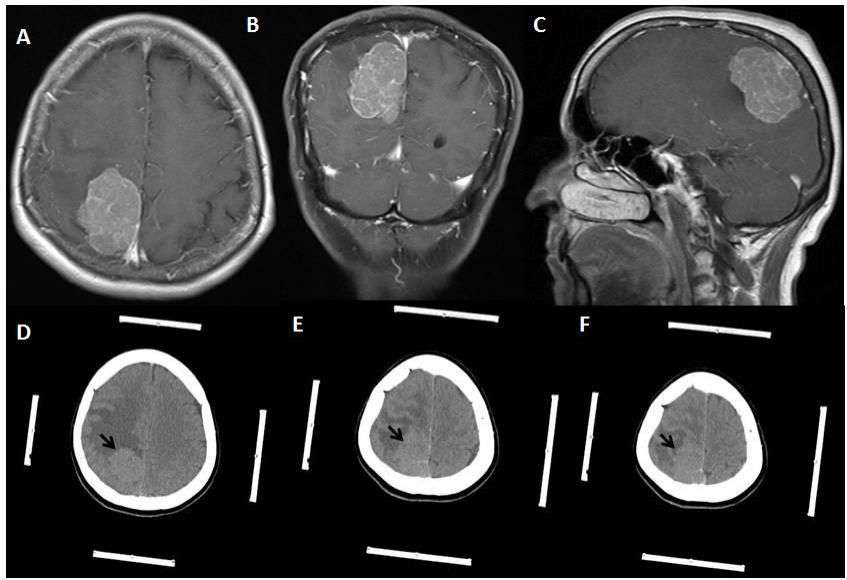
A, B, C: (Table 1: Case1, Grade 2 meningioma): T1 weighted gadolinium-enhanced axial magnetic resonance imaging (MRI) scans showing right frontoparietal mass located just next to the superior sagittal sinus and extended from right parietal lobe to the right frontal lobe’s precentral gyrus (motor cortex). D, E, F: Contrast-enhanced cranial tomography showing right frontoparietal mass located just next to the superior sagittal sinus and extended from right parietal lobe to the right frontal lobe’s precentral gyrus (motor cortex). The white strips at the sides of the images are the marker of the frame based the stereotaxic system*.
